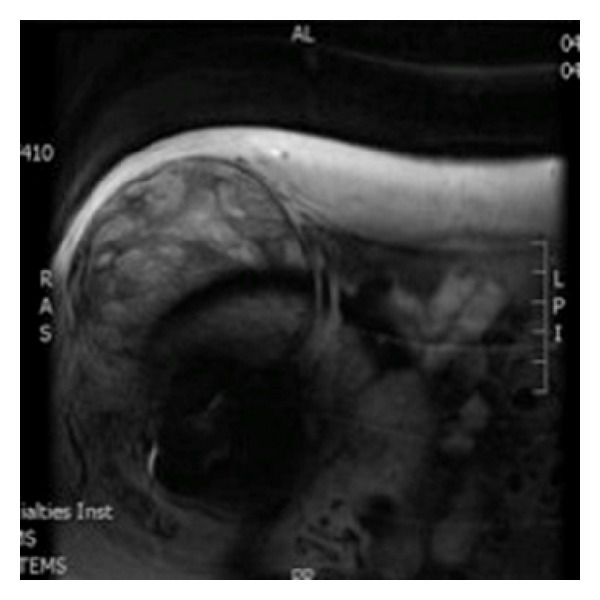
MRI showing 12.0 × 12.1 × 14.3 cm multilobulated soft tissue mass.
